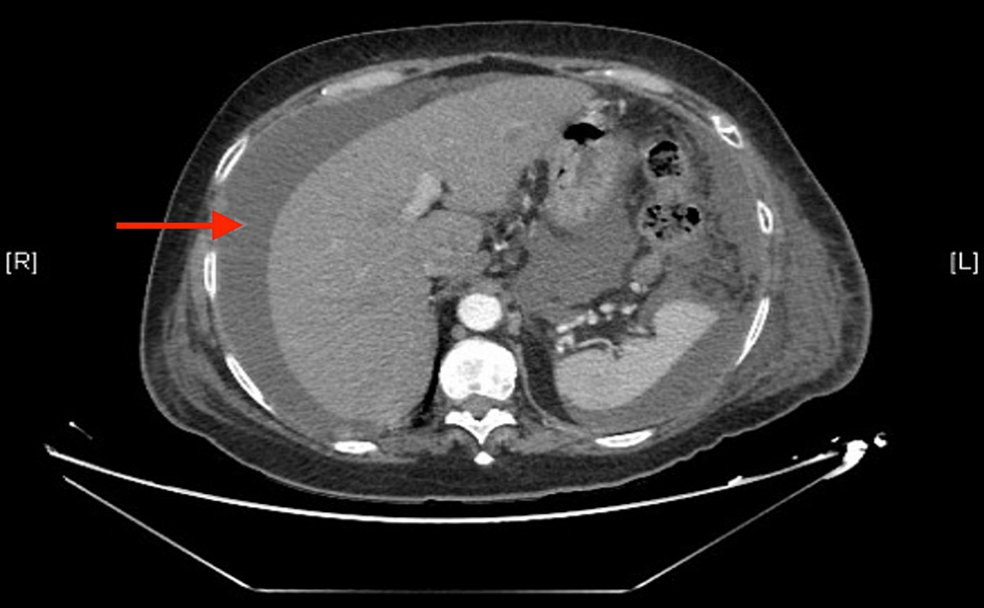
Sagital CT section showing abundant perihepatic, perisplenic and around bowel loops fluid. Red arrow point perihepatic, perisplenic and around bowel loops fluid.
